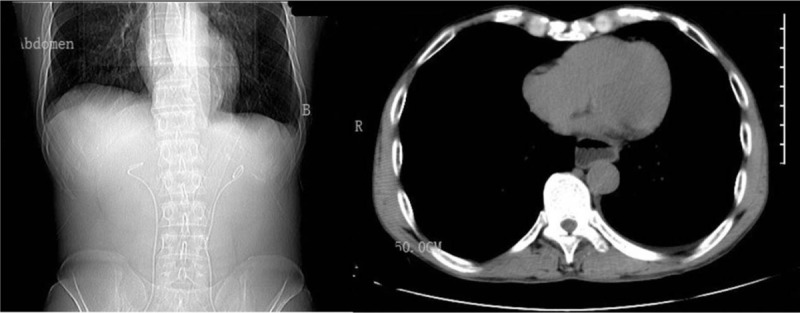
The esophagus computed tomography scan is normal before gastroscopy.
